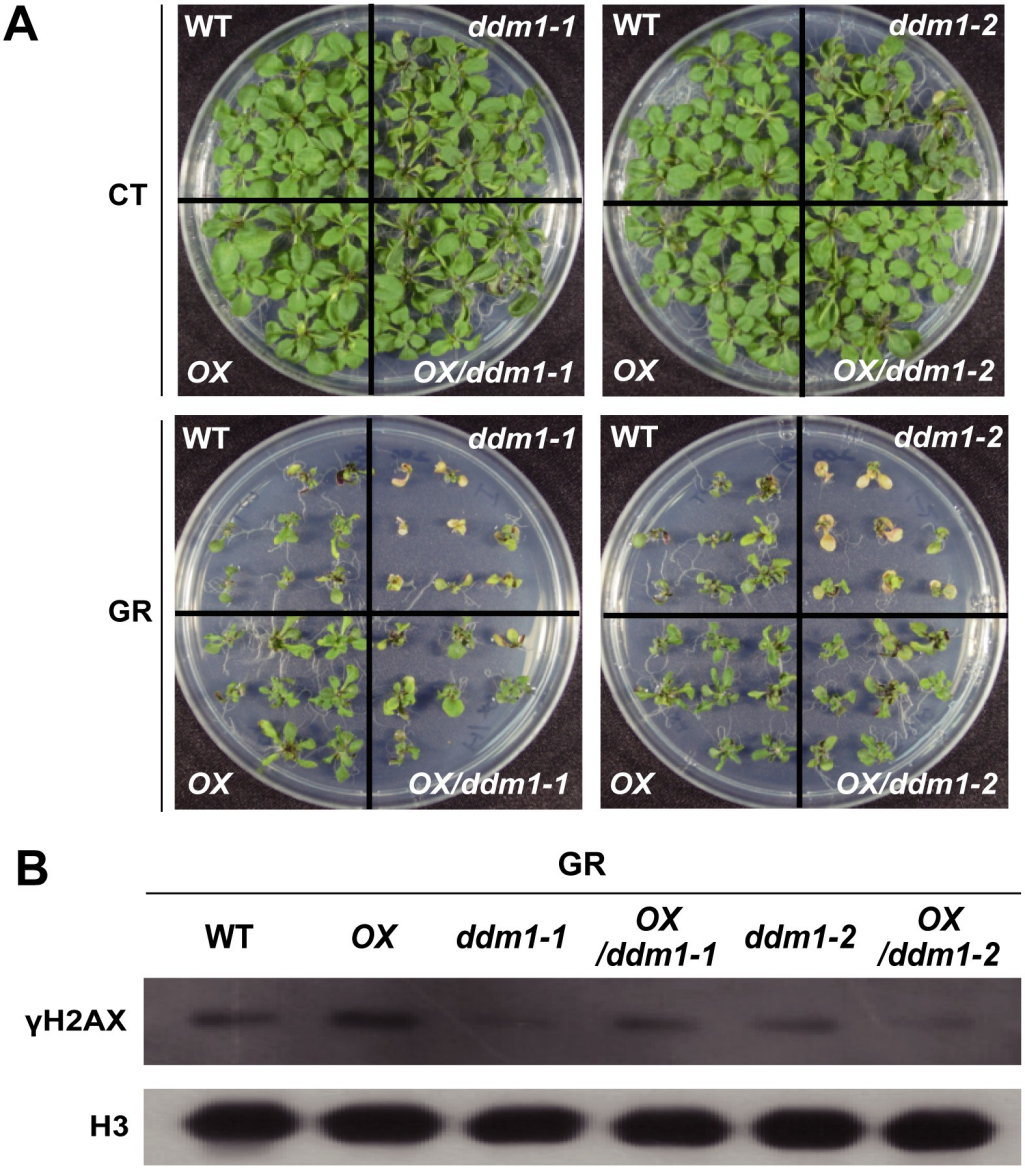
Complementation of *DDM1* mutation by overexpressing the full-length cDNA of *DDM1* in the ddm1 mutant is effective. (A) Phenotypes of transgenic lines overexpressing the *DDM1* cDNA in WT and *ddm1* mutant backgrounds in response to gamma irradiation. Seedlings were grown for 18 days after gamma irradiation at 200 Gy for 4 h. CT, control; GR, gamma radiation. (B) Immunoblot of γ-H2AX in transgenic lines overexpressing the *DDM1* cDNA in WT and *ddm1* mutant backgrounds after gamma irradiation at 200 Gy.
